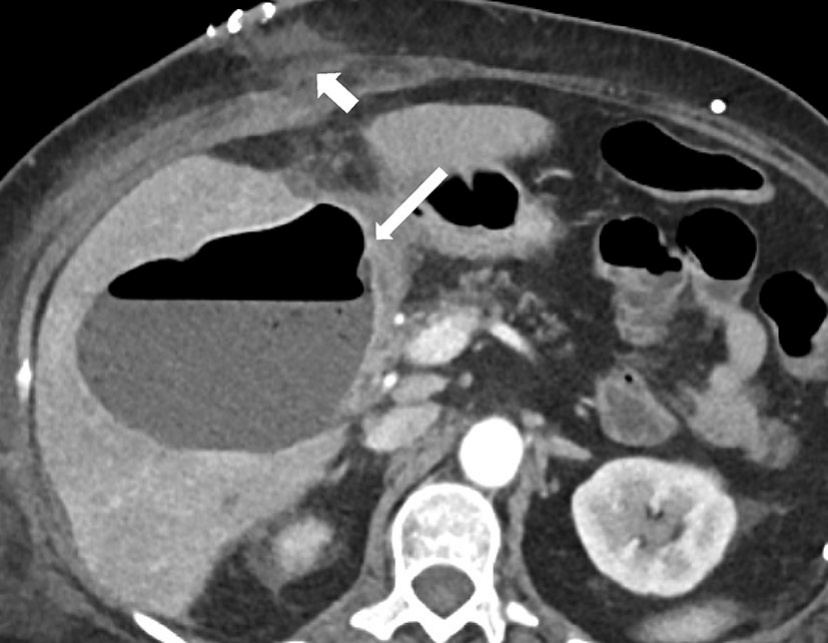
A 57-year-old woman with an abscess in the gallbladder fossa post-cholecystectomy. Axial postcontrast CT image demonstrates a thick-walled collection in the surgical bed, air-fluid level, and rim enhancement consistent with postsurgical abscess (long arrow). Postsurgical fat stranding and suture material are also seen in the anterior abdominal wall (short arrow)
